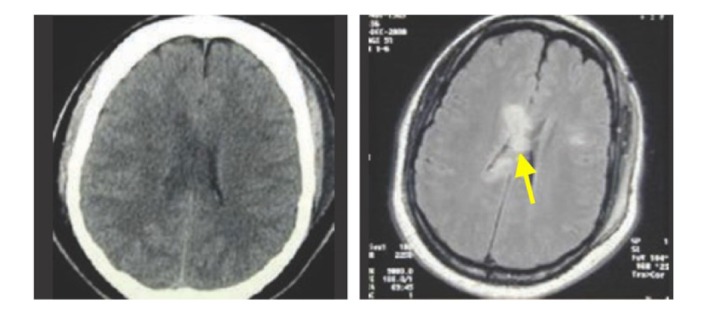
CT and MRI scans showing lesions compatible with diffuse axonal injury (DAI). The arrow signals a shear lesion at the corpus callosum.
